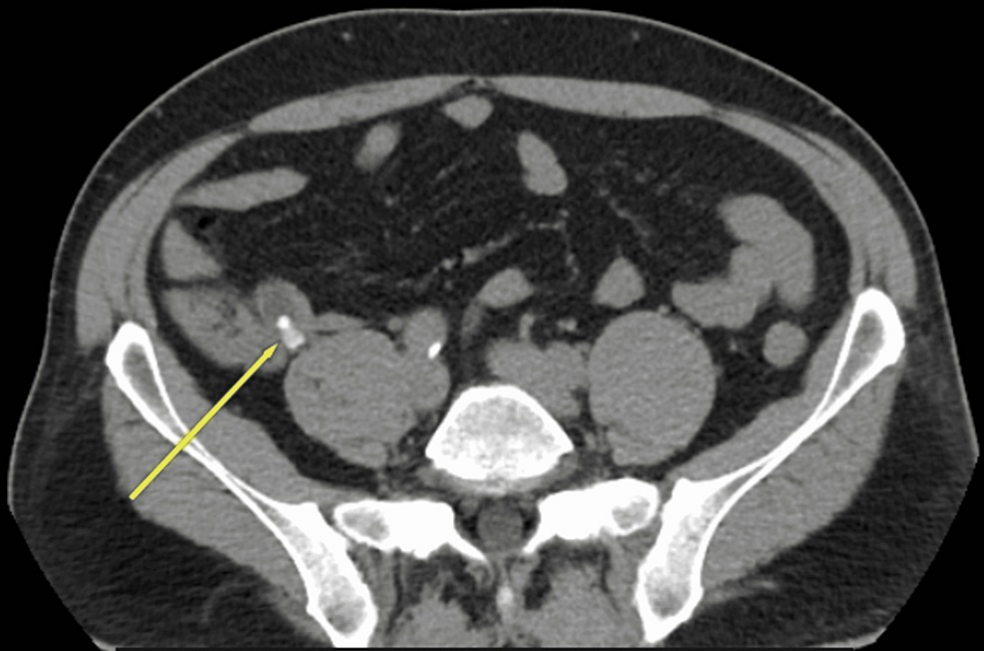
Axial view of abdominal CT, the yellow arrow indicates the obstruction of the appendix by appendicolith.
